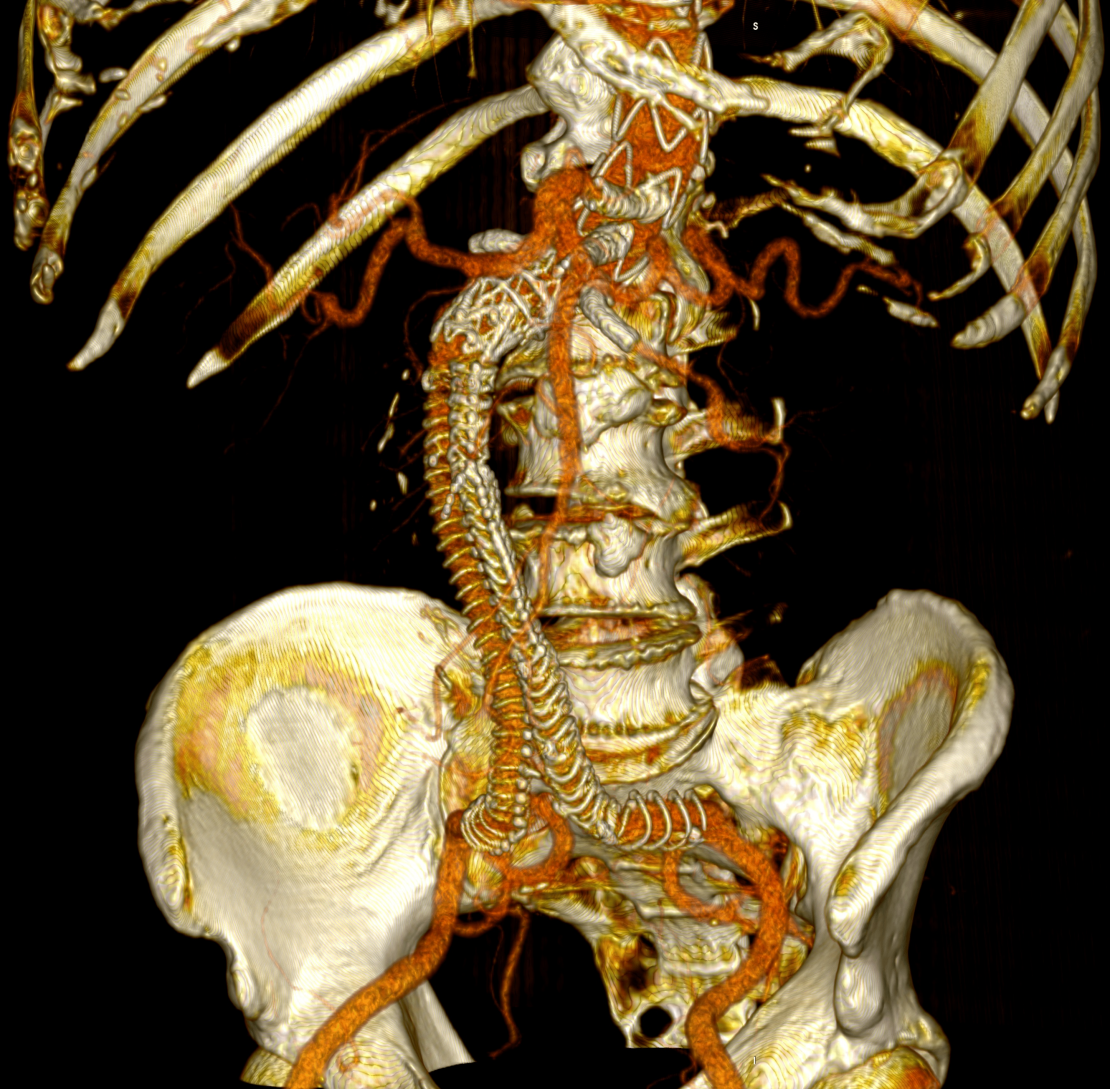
Postoperative 3D volume rendering of the fenestrated endograft reconstruction Three-dimensional volume-rendered reconstruction obtained in Horos using the standard soft-tissue preset. The image displays the postoperative appearance of the fenestrated endograft and visceral stents, extending from the descending thoracic aorta to the iliac bifurcation, acquired during the follow-up CTA one month after the procedure. The reconstruction shows patent visceral branches with well-perfused stents, absence of endoleak, and successful restoration of the previously disconnected left limb with adequate iliac perfusion.
